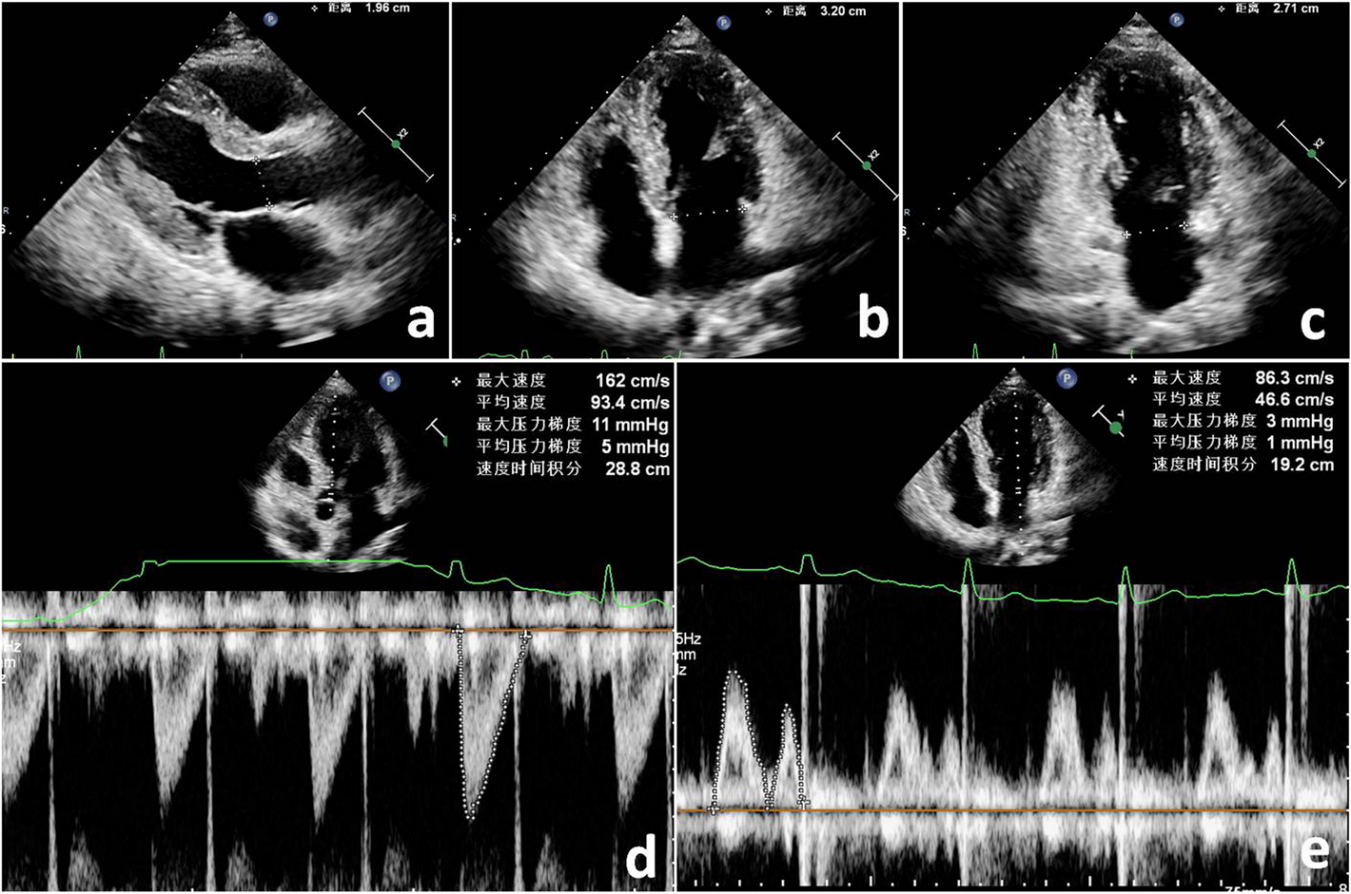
QPD calculations of MRvol assuming that the MA is circular geometry. a The diameter of LVOT was measured in the PLAX view, and the CSA_LVOT_ was derived as 0.785 d^2^. d LVOT pulsed Doppler was traced to obtain the VTI_LVOT_. Thus, SV_LVOT_ = 0.785 d^2^ × VTI_LVOT_ = 0.785 × 1.96^2^ × 28.8 = 86.85 ml. b and c The diameter of MA was measured in the A4C and A2C view. The CSA_A4C_ was derived as 0.785 × 3.2^2^, and CSA_A2C_ was derived as 0.785 × 2.71^2^. e MA inflow pulsed Doppler was traced to obtain the VTI_MA._ Thus, SV_A4C_ = 0.785 × 3.2^2^ × 19.2 = 154.33 ml, and SV_A2C_ = 0.785 × 2.71^2^ × 19.2 = 110.69 ml. In this example of MR, MRvol by QPD-MA_A4C_ = SV_A4C_ - SV_LVOT_ = 154.33–86.85 = 67.48 ml, and MRvol by QPD-MA_A2C_ = 110.69–86.85 = 23.84 ml
